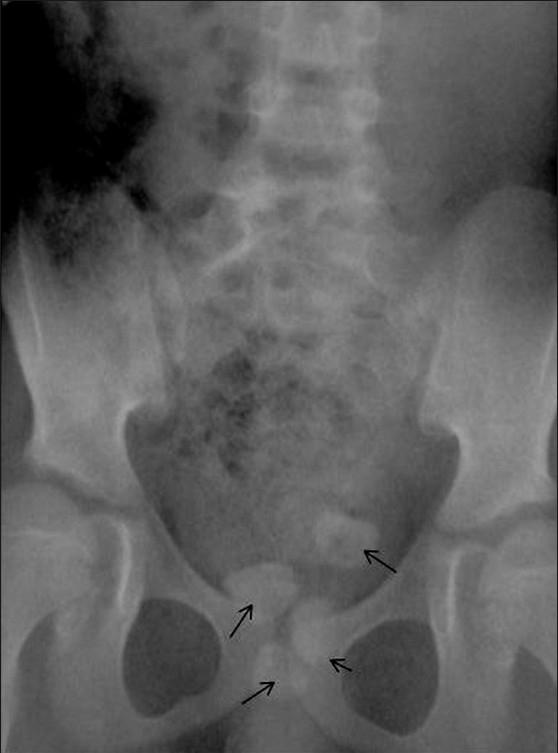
Anteroposterior radiograph of the abdomen and pelvis reveals multiple radiodense calculi (arrows) in the pelvis, overlying the symphysis pubis
